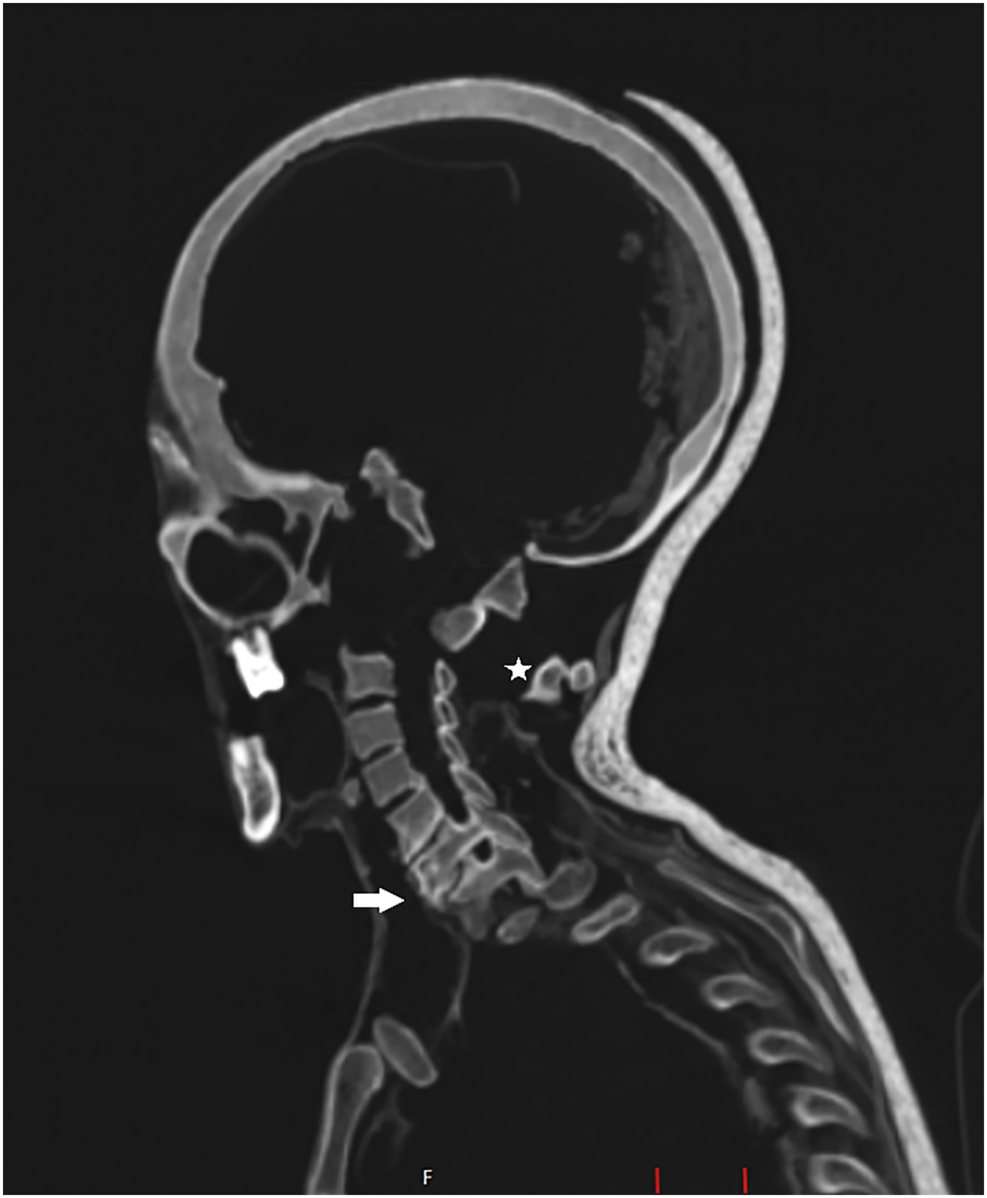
Sagittal multi-planar reconstruction of a CT data set showing the skull and the cervical spine of individual A. Remnants of the shrunken brain are visible in the posterior cranial fossa. The lower cervical and upper thoracic spine reveals degenerative changes (white arrow). The cranio-cervical junction depicts severe displacement of the atlas and axis (asterisk).
